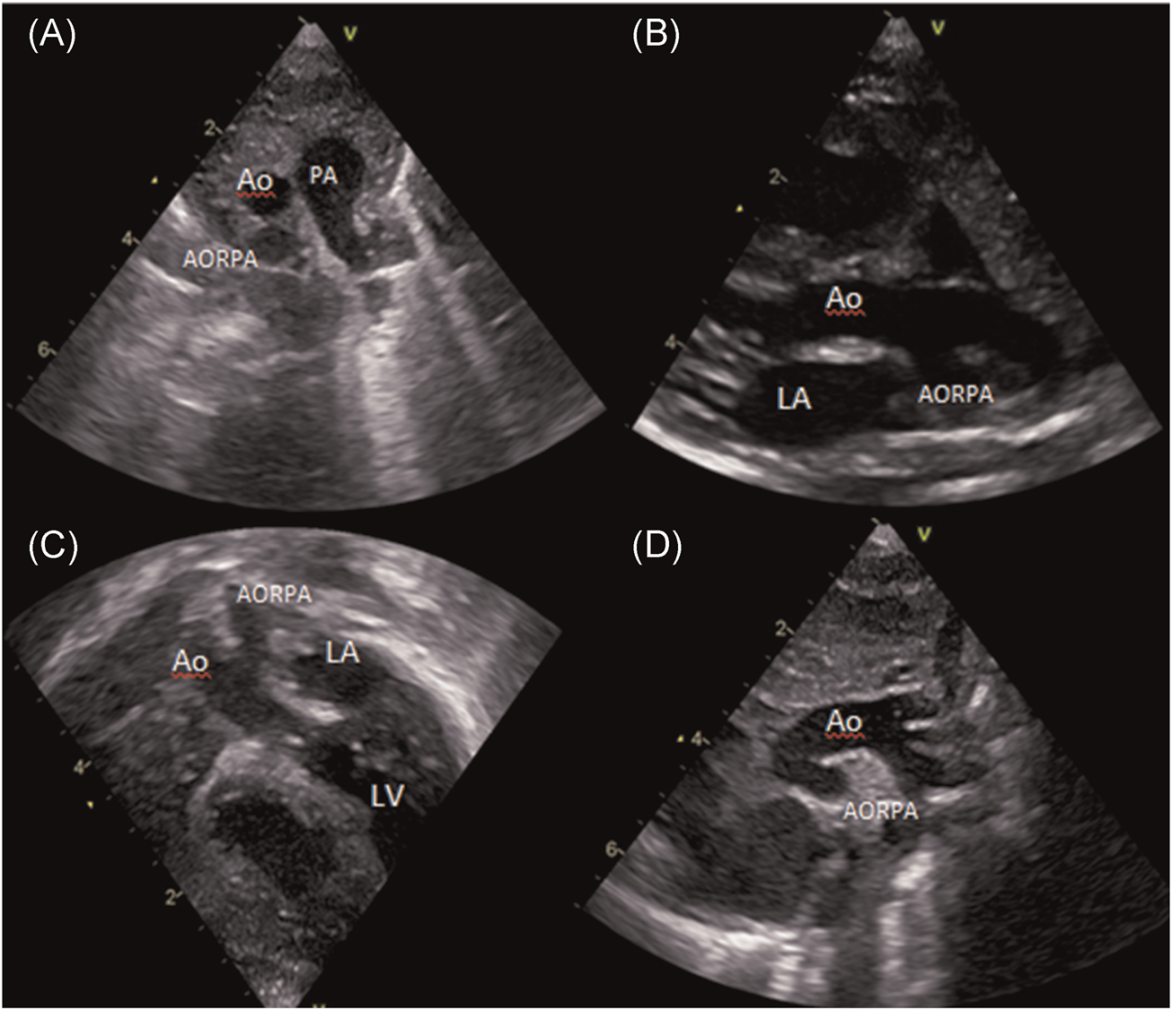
The preoperative echocardiogram. (A) Short‐axis parasternal view showing no continuity between the main pulmonary artery and the right pulmonary artery, (B) long‐axis parasternal view, (C) 5 chamber view, (D) suprasternal view all showing abnormal vessel coming out of the ascending aorta. Ao, aorta; AORPA, anomalous origin of right pulmonary artery from aorta; LA, left atrium; LV, left ventricle; PA, pulmonary artery
